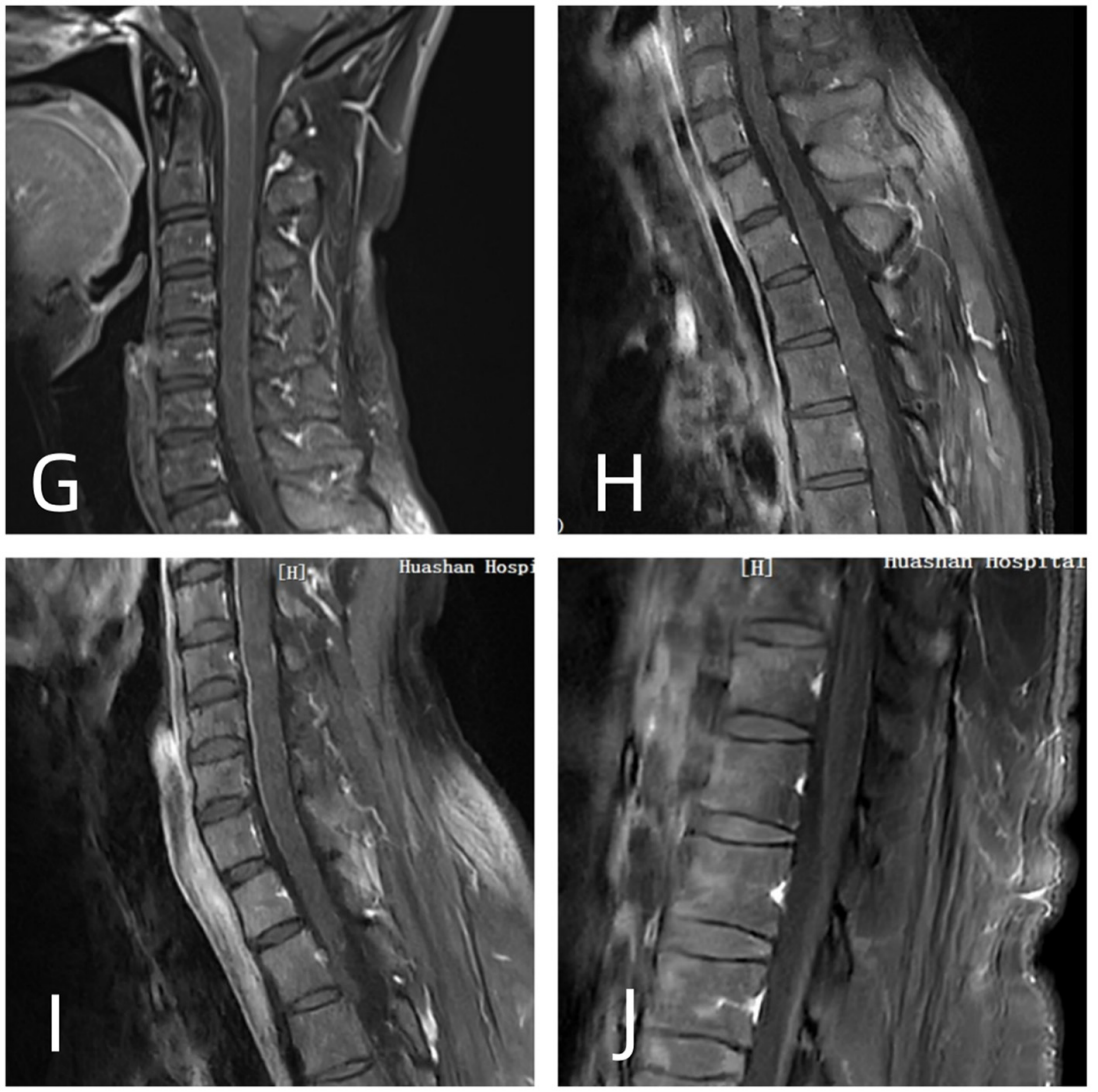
(G, I) T1-weighted contrast-enhanced (CE-T1WI): linear enhancement of the cervical spinal cord pia mater. (H) CE-T1WI: linear enhancement of the pial membrane in the thoracic spinal cord (J) CE-T1WI: leptomeningeal enhancement around the conus.
